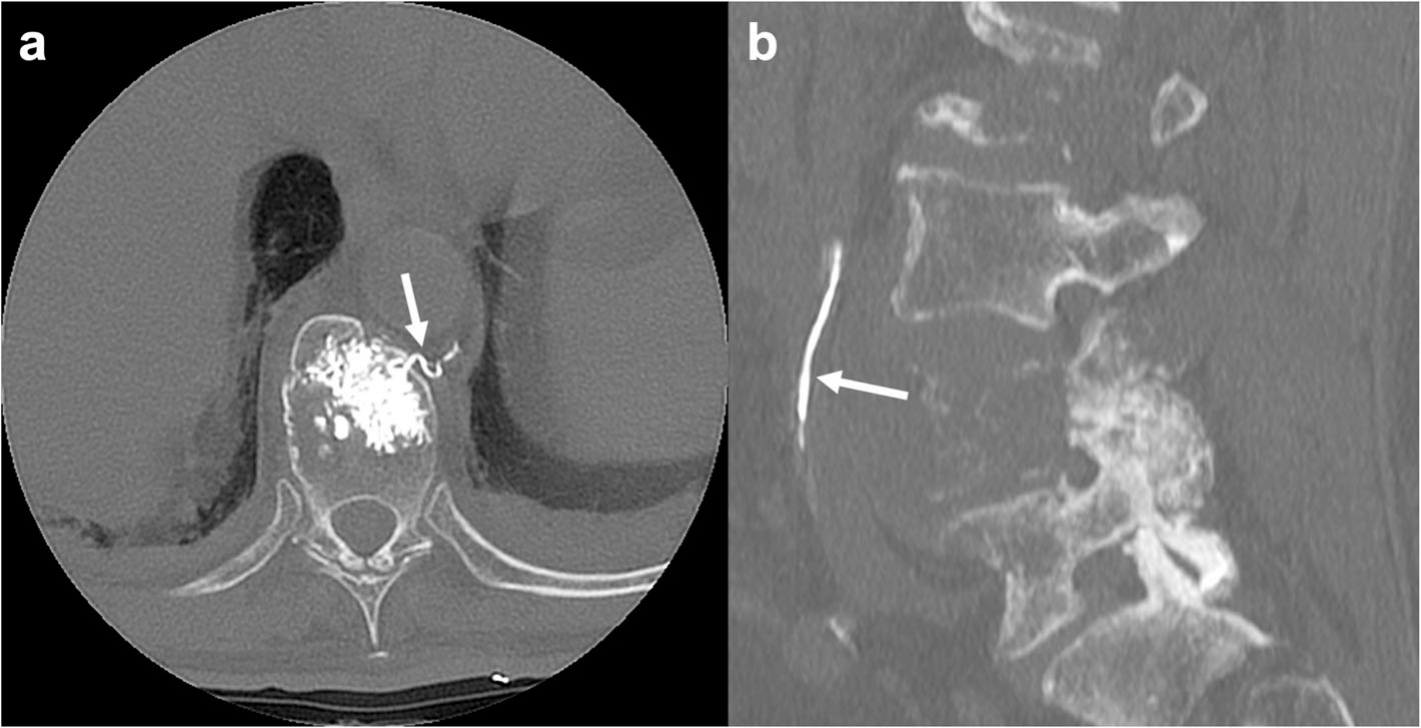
Polymethylmethacrylate migration through veins. Axial (a) and sagittal (b) unenhanced CT presenting cement migration through an external anterior plexus vein (arrow in a) to the inferior vena cava (arrow in b)
